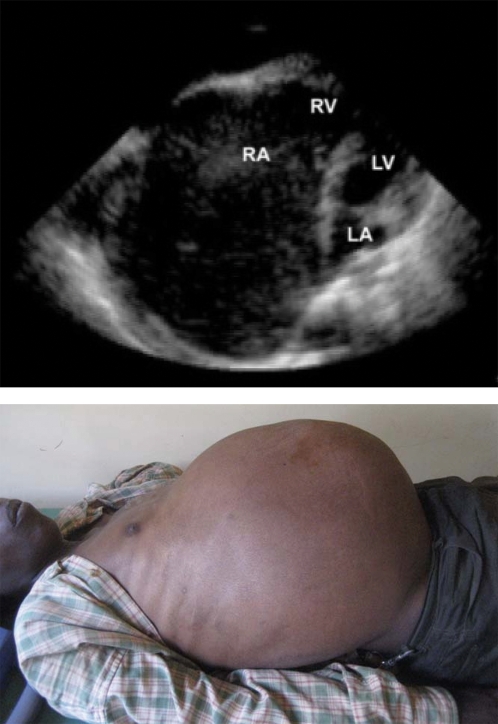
Top, echocardiogram in a 25-y-old man with predominantly right ventricular EMF from eastern Rwanda. Apical four-chamber view. Note the marked dilatation of the right atrium. RV = right ventricle, RA = right atrium, LV = left ventricle, LA = left atrium. Bottom, massive ascites in the same patient.
